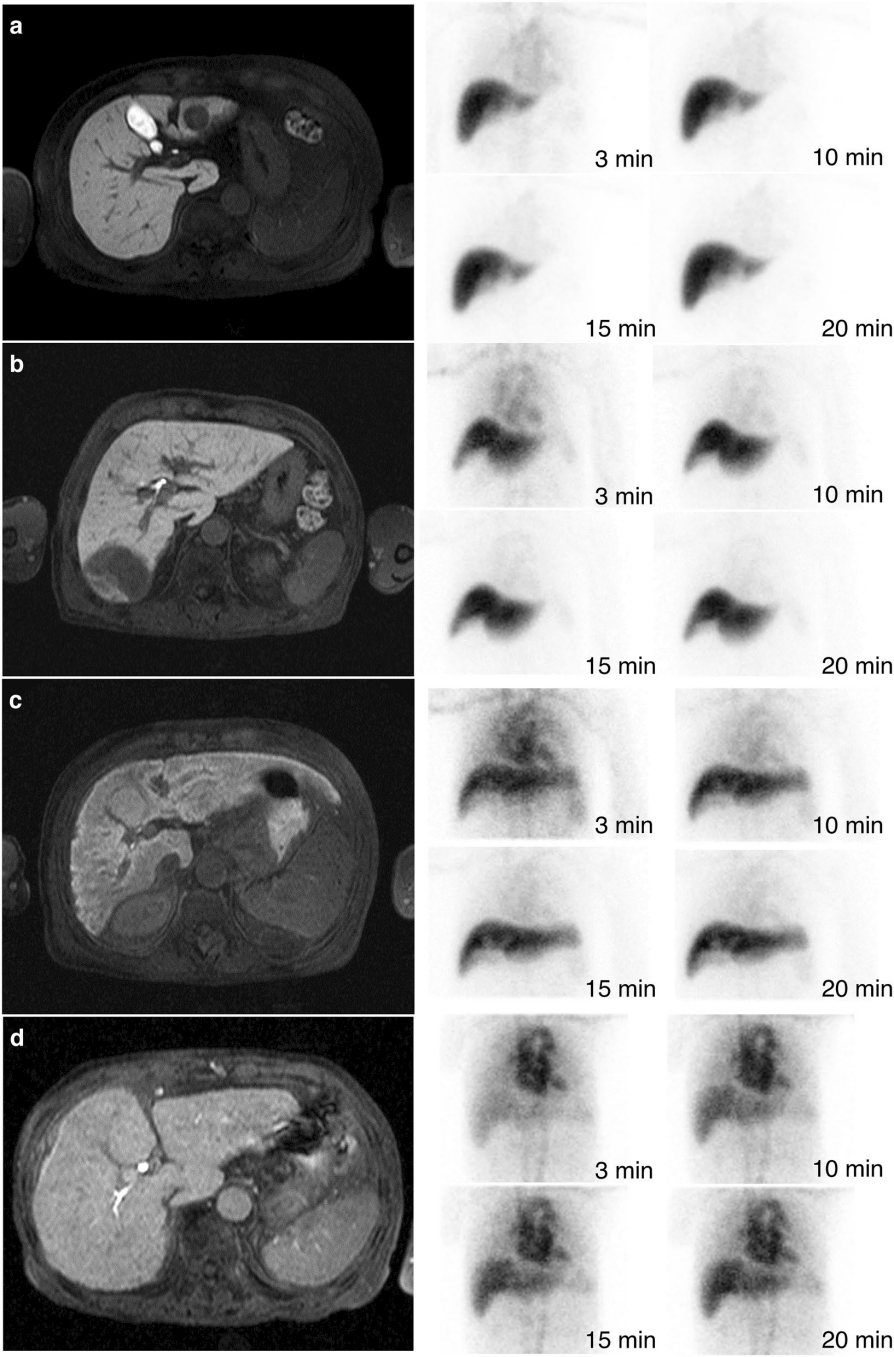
Reference images of various imaging-based clinical stages. The left and right image in figures are the hepatobiliary-phase images in Gd-EOB-DTPA-enhanced MRI and the planer images in ^99m^Tc-GSA liver scintigraphy, respectively. (a) Images of Normal stage. The LSC, eLHL15, LHL15, HH15, and ALBI score were 0.487, 0.95, 0.95, 0.61, and –2.899 (Grade 1), respectively. (b) Images of Mild stage. The LSC, eLHL15, LHL15, HH15, and ALBI score were 0.375, 0.90, 0.90, 0.61, and –2.756 (Grade 1), respectively. (c) Images of Moderate stage. The LSC, eLHL15, LHL15, HH15, and ALBI score were 0.257, 0.85, 0.84, 0.69, and –1.426 (Grade 2), respectively. (d) Images of Severe stage. The LSC, eLHL15, LHL15, HH15, and ALBI score were 0.114, 0.78, 0.65, 0.88, and –2.150 (Grade 2), respectively.
